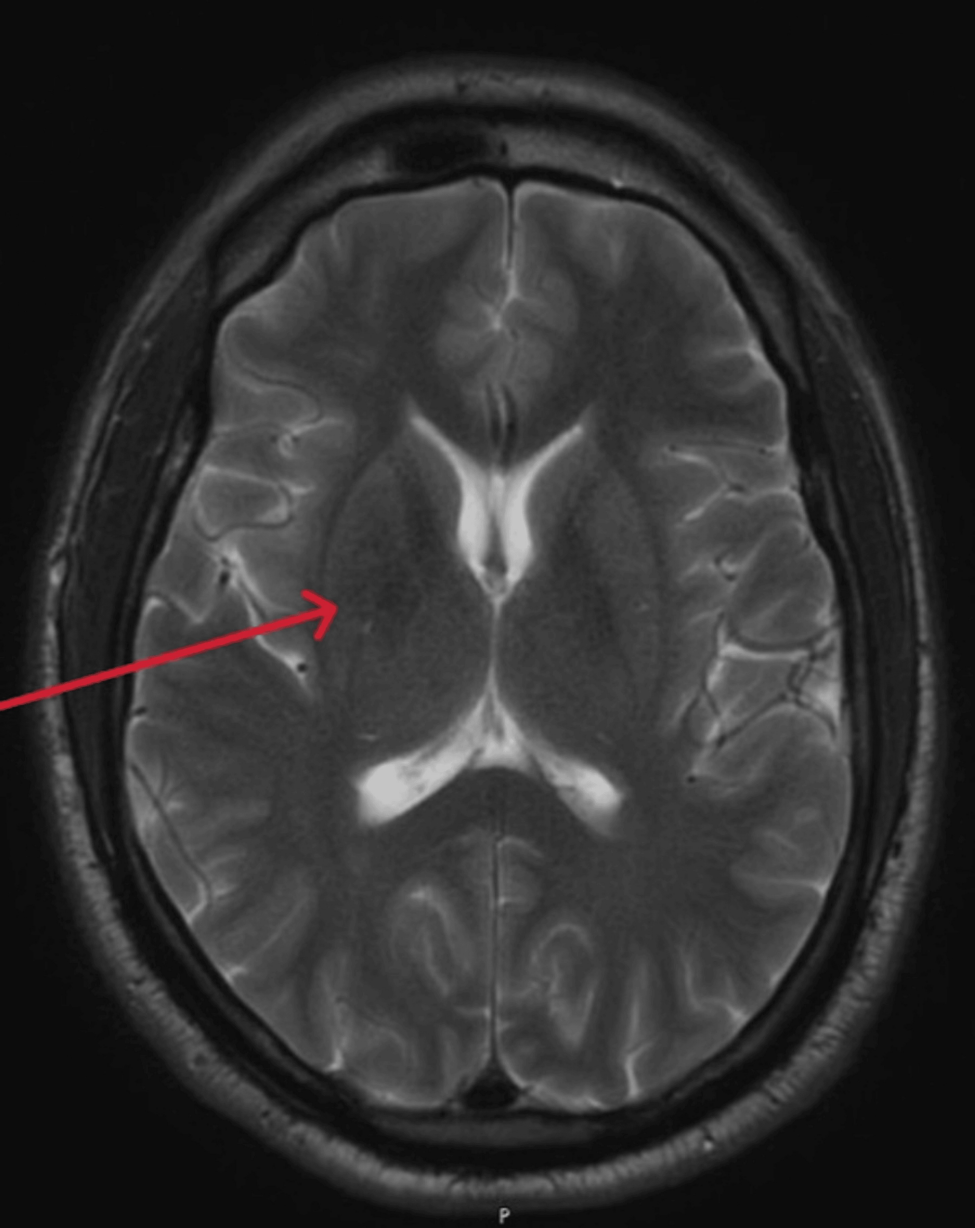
T2-weighted magnetic resonance imaging showing subtle hypointensity noted in the right basal ganglia (red arrow)
